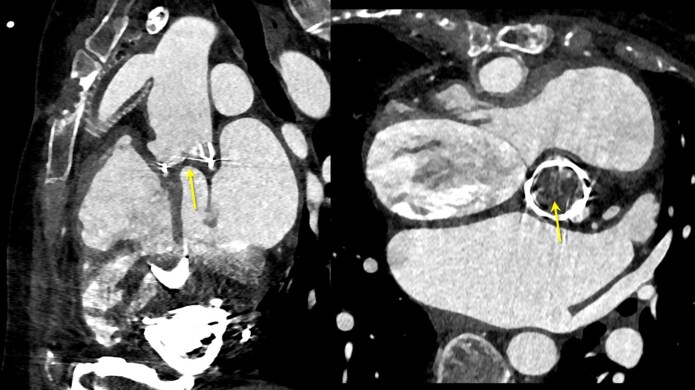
An example of an intra-aortic thrombus on a CT scan (arrow).
